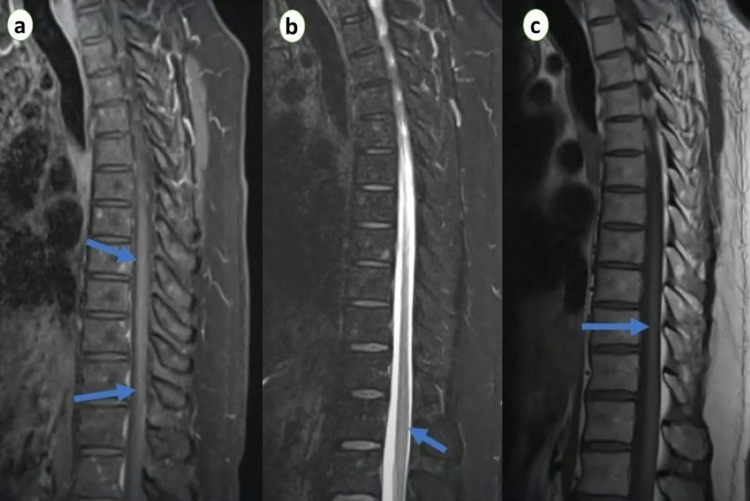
MRI thoracic spine: (a) Sagittal T1 sequence with gadolinium contrast. (b) Sagittal T1 sequence of the thoracic spine. (c) Sagittal T2 sequence showing a high signal intensity starting from the T2 level.
